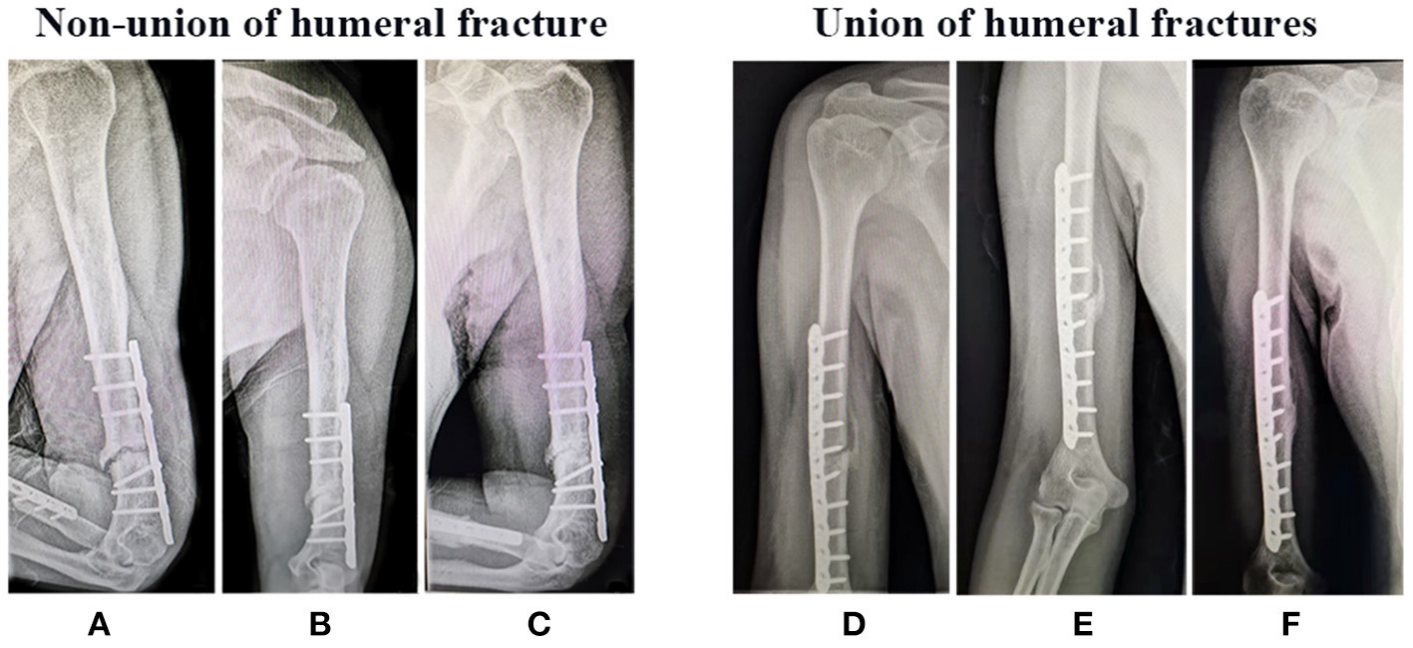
Non-union and Union of humeral fractures after surgery, respectively. X-ray examination (A,D) 1 month, (B,E) 3 month, and (C,F) 9 month after surgery.
